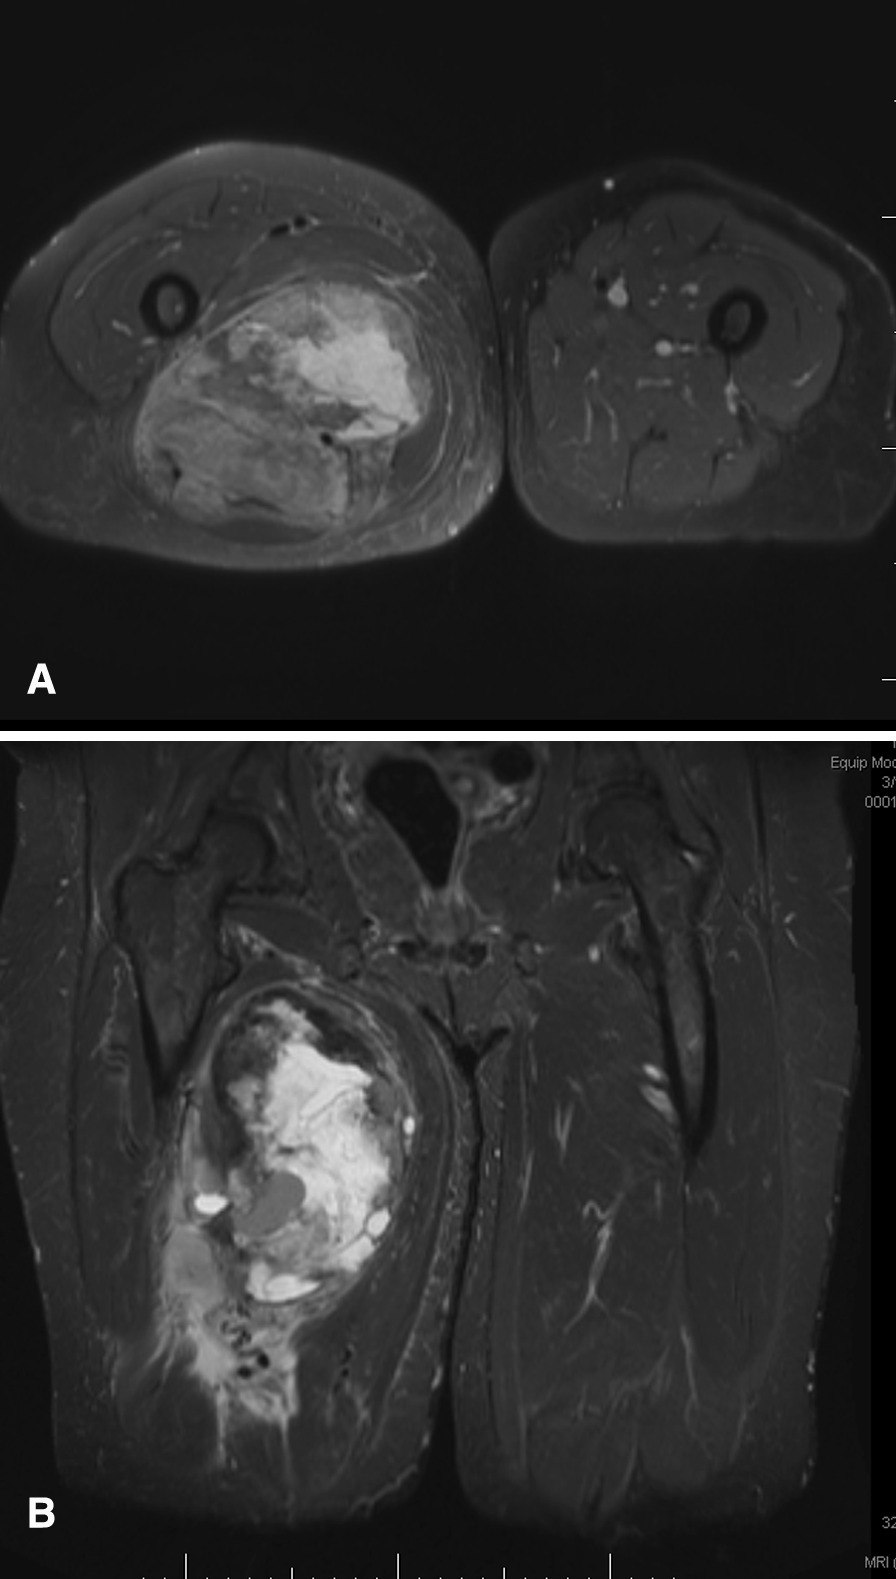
Pre-treatment MRI images. A Axial PD fat suppressed MR image of the right thigh demonstrates a heterogeneous soft tissue mass measuring 13.5 × 11.4 cm in transverse and AP dimension; B Coronal stir (short T1 inversion recovery) MR image of a heterogenous soft tissue mass measuring 20.8 × 11.3 cm in craniocaudal and transverse measurement
